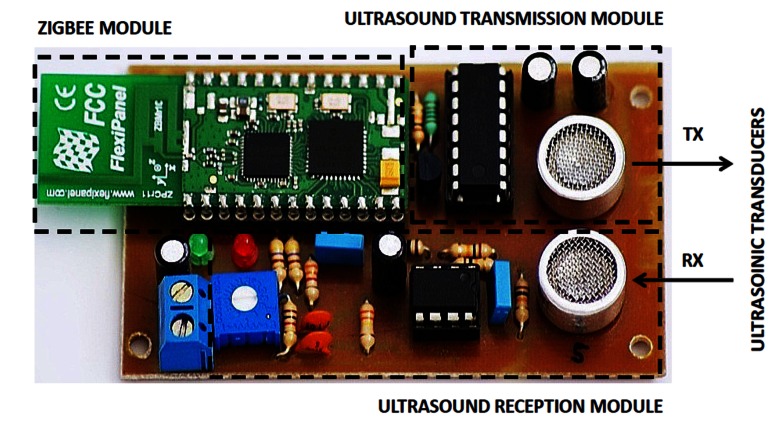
Picture of a TELIAMADE node. The regions identified by dashed line show the hardware components of the radio module and the signal conditioning modules for transmission and reception of ultrasonic signal.
